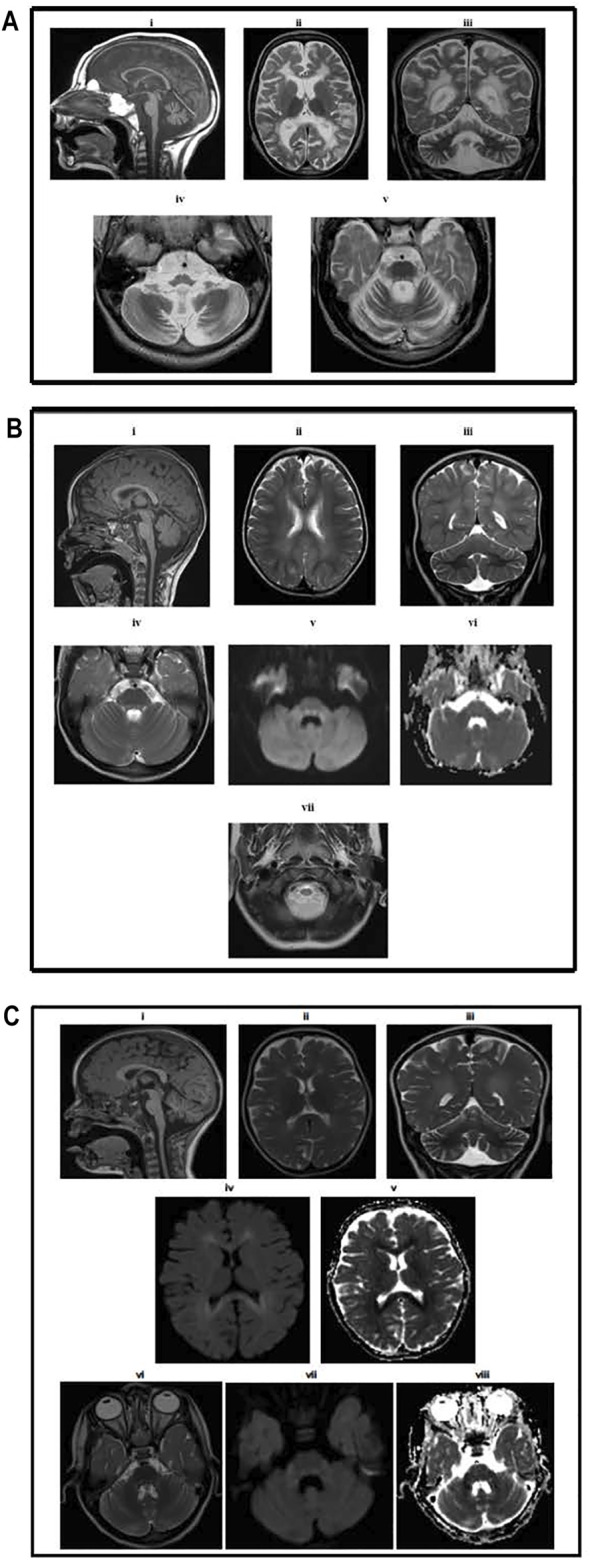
(A) Brain MRI results for Patient II-1. MR examination of the brain at the age of 5. (i) T1-weighted image showing atrophy of the corpus callosum and cerebellum and medulla oblongata and upper cervical spinal cord with normal-sized pons and midbrain. Axial (ii) and coronal (iii) T2-weighted images demonstrating extensive high signal intensity in the periventricular and deep white matter with relative sparing of the subcortical white matter. The cerebral and cerebellar atrophy is evident by the large ventricular system, sulci, and folia. Axial T2-weighted images at the level of the medulla oblongata (iv) showing medullary atrophy and at the level of pons (v) showing signal alteration, mainly involving the dorsal and peripheral parts of the pons. (B) Brain MRI result for Patient II-8. MR examination of the brain at the age of 2 years. (i) T1-weighted image showing the normal corpus callosum, cerebellum, and brainstem. The upper cervical spinal cord is atrophic. Axial (ii) and coronal (iii) T2-weighted images demonstrating mild high signal intensity in the periventricular and deep white matter, sparing the subcortical white matter. There is no cerebral or cerebellar atrophy. Axial T2-weighted (iv), diffusion-weighted (v) images, and the apparent diffusion coefficient map (vi) at the level of pons showing signal alteration mainly involving the dorsal and peripheral parts of the pons with diffusion restriction in the dorsal pons (vii). (C) Brain MRI results for patient IV-1. MR examination of the brain at the age of 18 months. (i) T1-weighted image showing mild atrophy of the cerebellum normal corpus callosum, brainstem, and upper cervical spinal cord. Axial (ii) and coronal (iii) T2-weighted images demonstrating mild high signal intensity in the periventricular and deep white matter, sparing the subcortical white matter. There is mild cerebral and cerebellar atrophy. Axial diffusion-weighted (iv) images and apparent diffusion coefficient map (v) at the level of the lateral ventricles showing diffusion restriction in the periventricular and deep white matter. Axial T2-weighted (vi), diffusion-weighted (vii), and apparent diffusion coefficient map (viii) showing signal alteration involving the dorsal pons with diffusion restriction.
